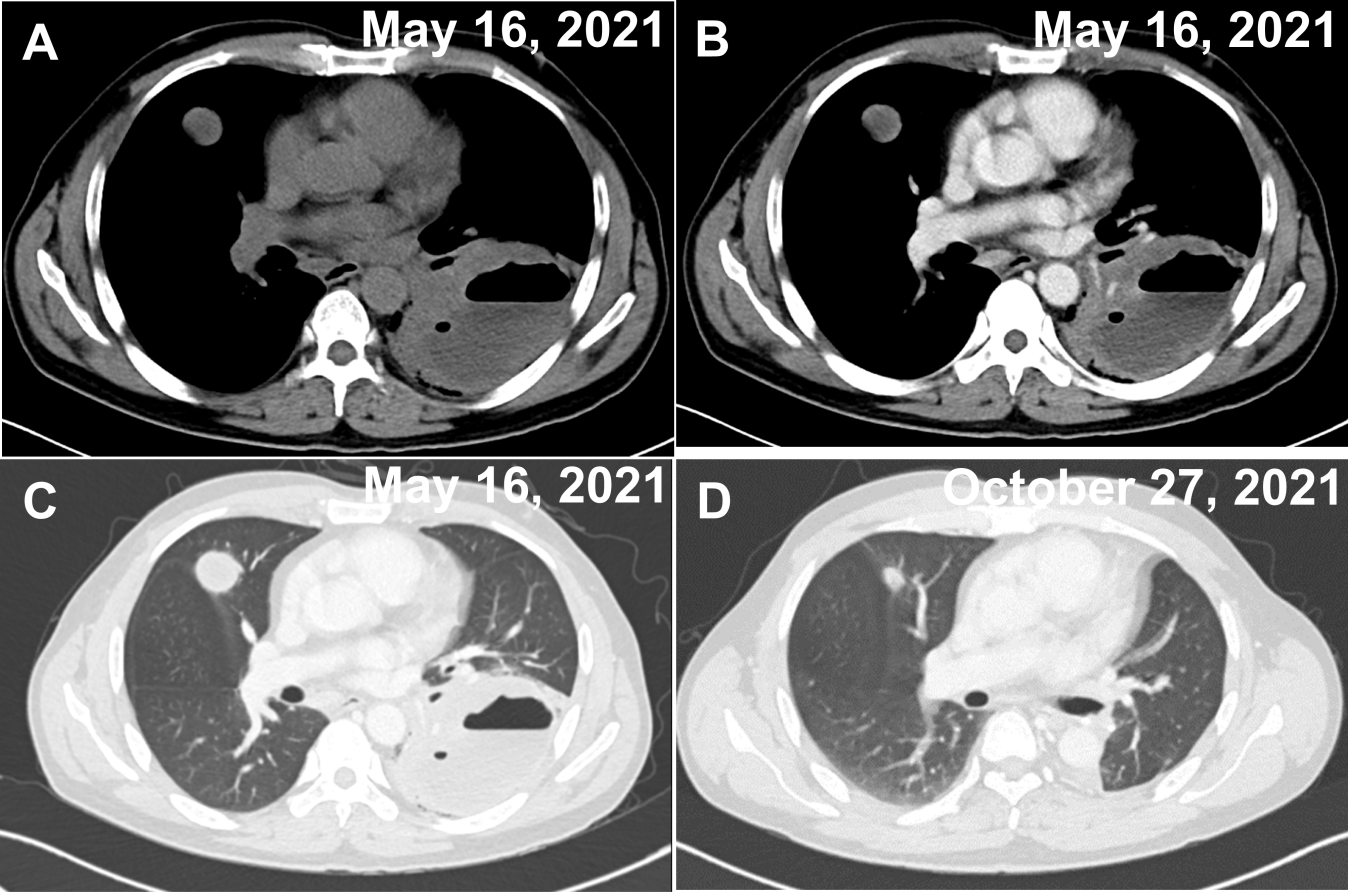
Two CT images of the chest on admission and after treatment. (A–C) A cavernous and fluid-flat mass in the lower lobe of the left lung and multiple nodules in the upper lobe of both lungs (the largest nodule is shown in the figure), both of which show mild circumferential enhancement on enhancement scans. (A) Unenhanced period. (B) Venous phase. (C) Pulmonary window. (D) Postoperative changes in the left lung, with significantly smaller nodules in the upper lobes of both lungs (largest nodule in the figure) than before.
